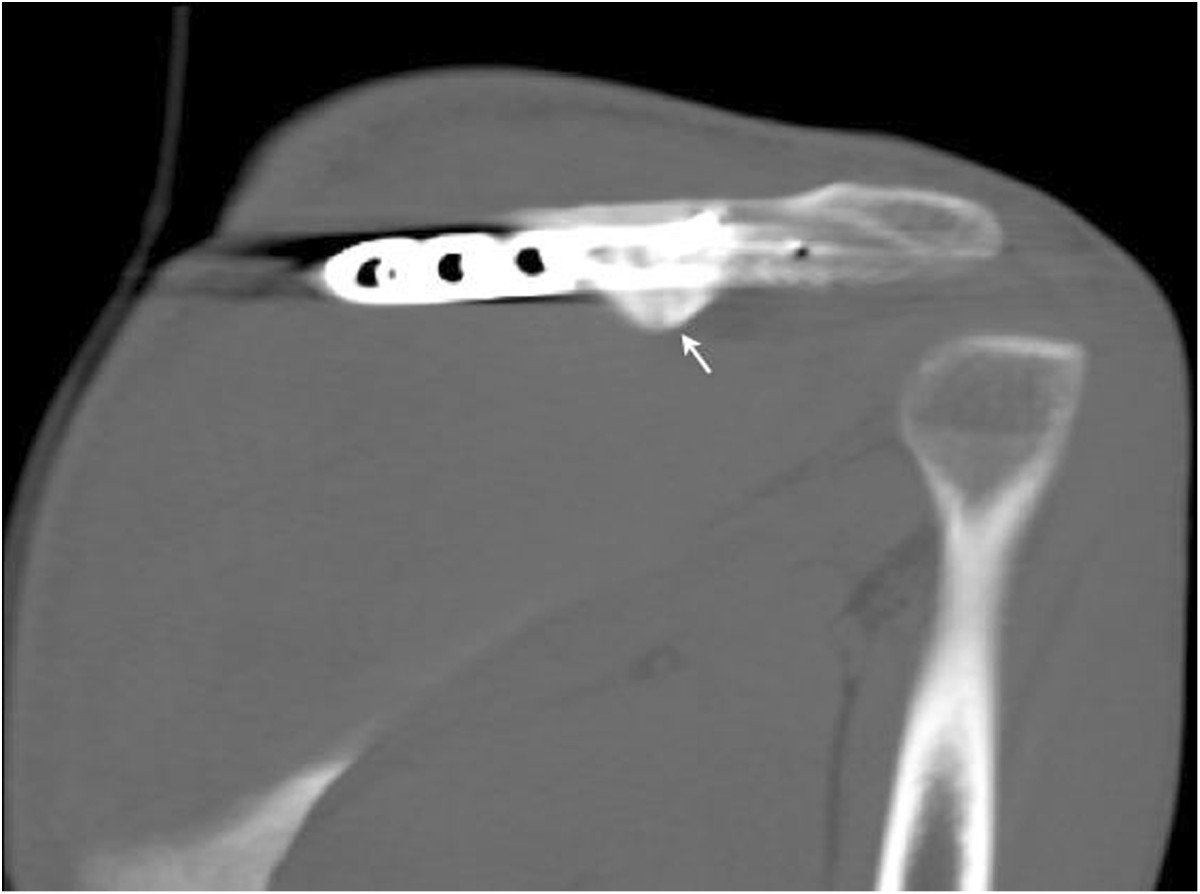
Postoperative CT. Oblique coronal reconstruction CT images of the left shoulder performed 5 months after the surgery demonstrating fracture consolidation.
